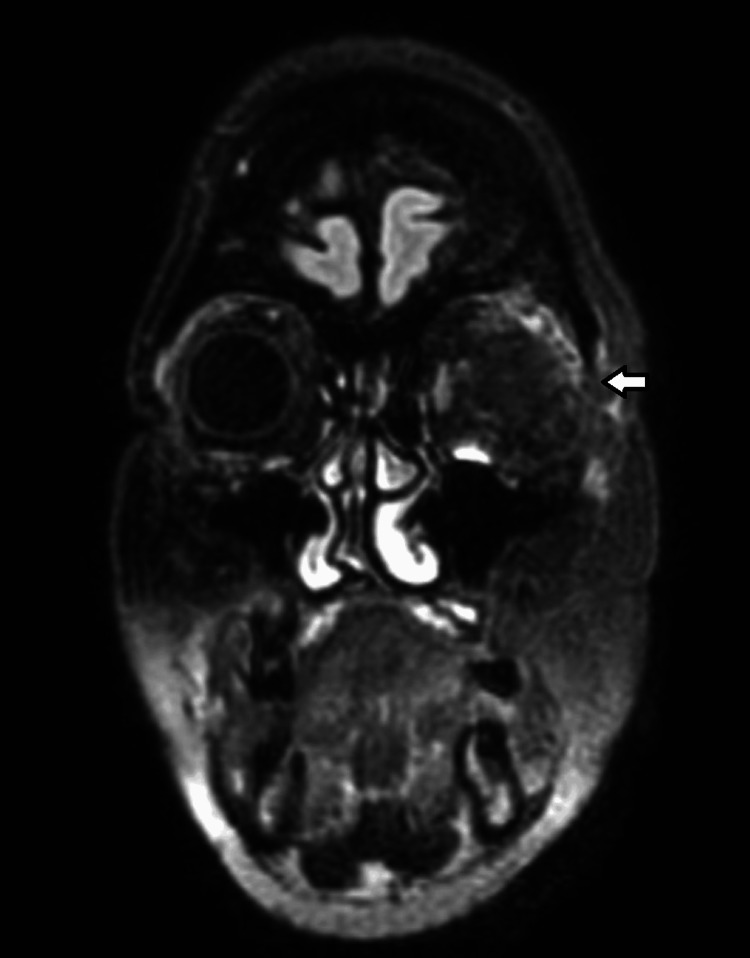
Pre-operative MRI FLAIR scan Meningioma of the left sphenoid wing, with projection into the temporal fossa and orbit, with diffuse meningeal extension to the temporal squama and left frontal bone. It reduces the left orbit, which compresses the muscles and optic nerve in the posterior part and causes proptosis.
